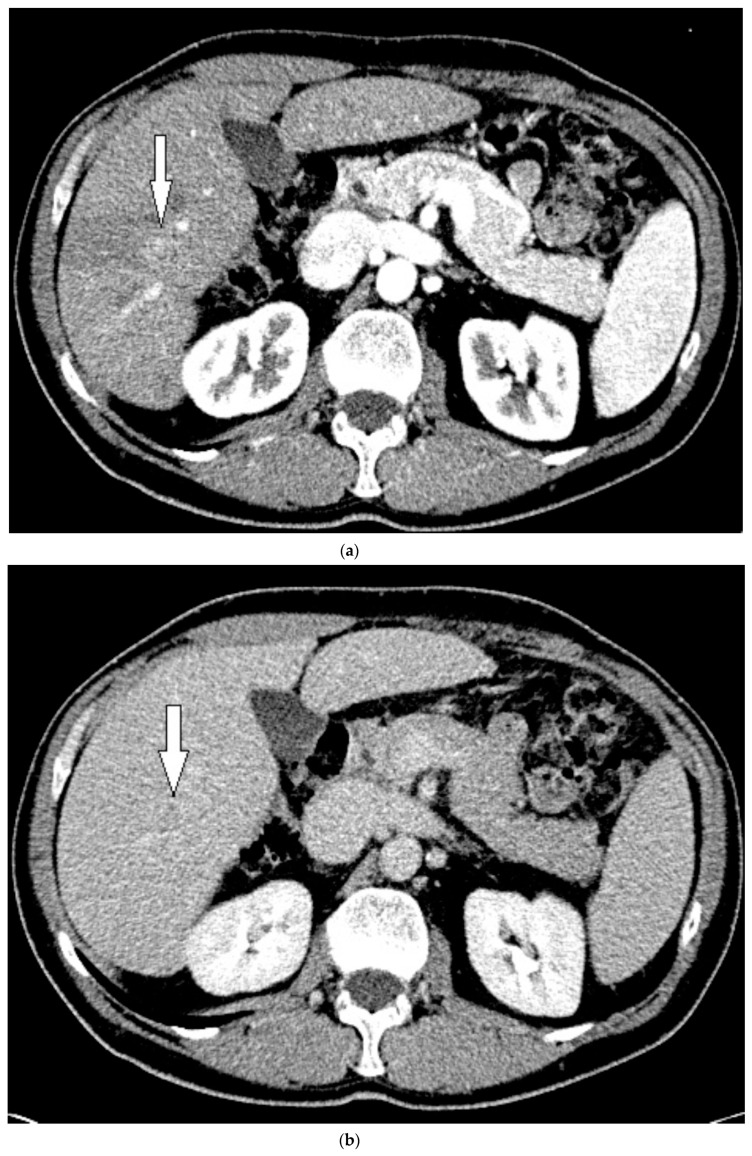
Patient 1 CT images using liver windows to highlight the subtle lesion easily seen on contrast-enhanced ultrasound (CEUS) (white arrow): (a) late arterial phase axial contrast-enhanced CT; (b) 3 min delayed phase.
